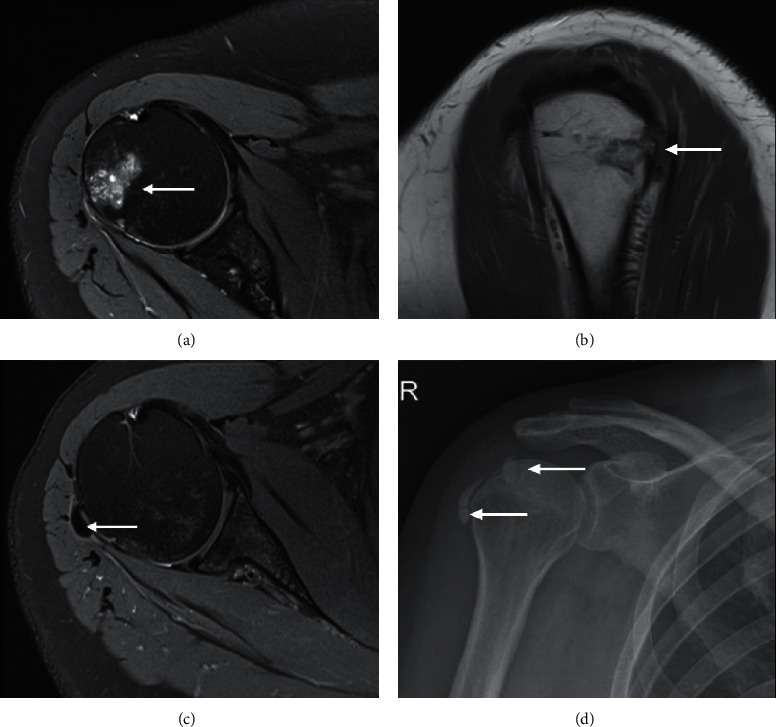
Calcific tendinitis in a 40-year-old female presenting with persistent shoulder pain. Axial PDFS (a) demonstrating marrow oedema signal in the humeral head at the greater tuberosity at teres minor tendon footprint. Sagittal PDFS (b) demonstrating corresponding cortical erosion. The MRI performed 5 months previously at the comparable plane (c) showed globular hypointense structure within the teres minor tendon footprint (arrow), confirming intraosseous migration of the calcific tendinitis in the most recent images. AP radiograph of the right shoulder (d) performed at the time of initial presentation also demonstrated calcium deposition in rotator cuff tendons. The calcium deposit is not well appreciated in the more recent study given the acute resorptive phase.
